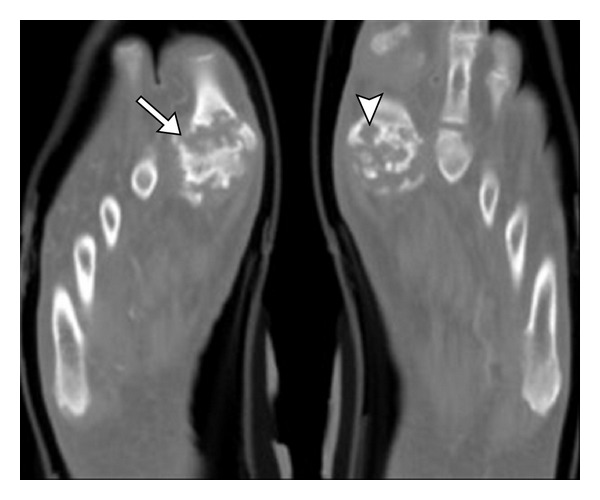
Advanced erosive gout on CT. Axial CT images of bilateral 1st MTP joints demonstrate severe erosive changes (arrow) related to chronic gout with intra-articular erosions and subchondral deposits (arrowhead). Note the preservation of bone density adjacent to erosions, a feature of gout.
